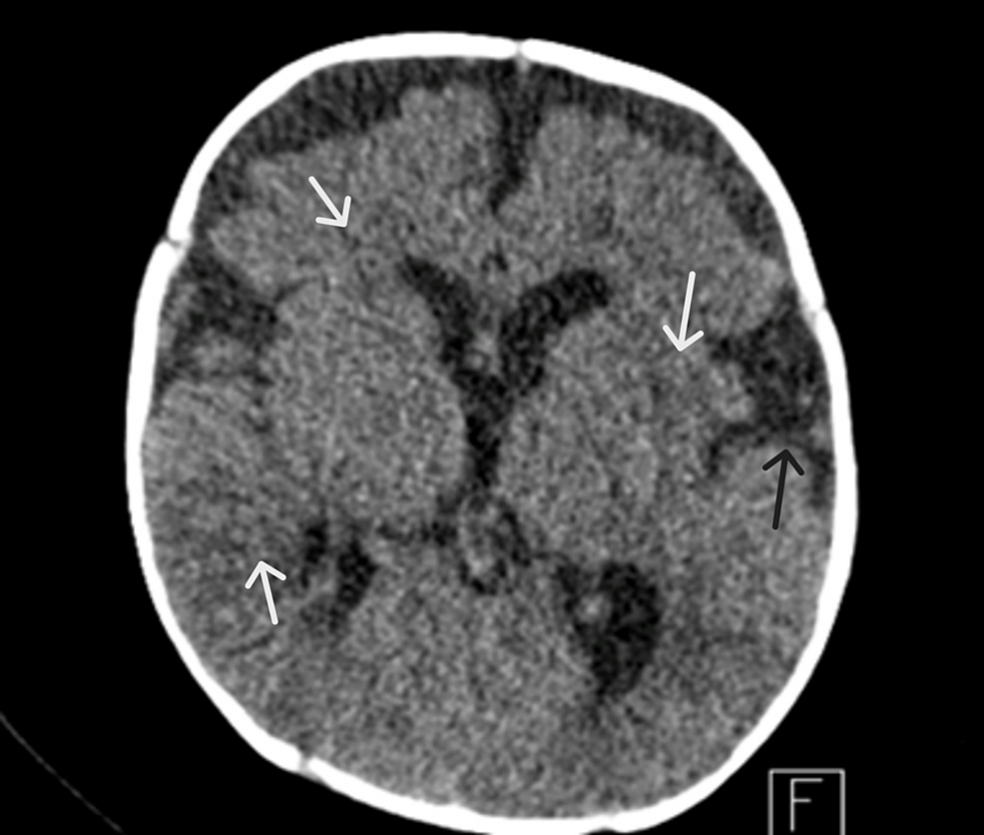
Repeat brain CT White arrows: Interval development of bilateral diffuse deep and periventricular white matter hypodensities; Black arrow: Minimal interval increases in the dilated extra-axial subarachnoid spaces, suggesting the presence of increased intracranial pressure.
